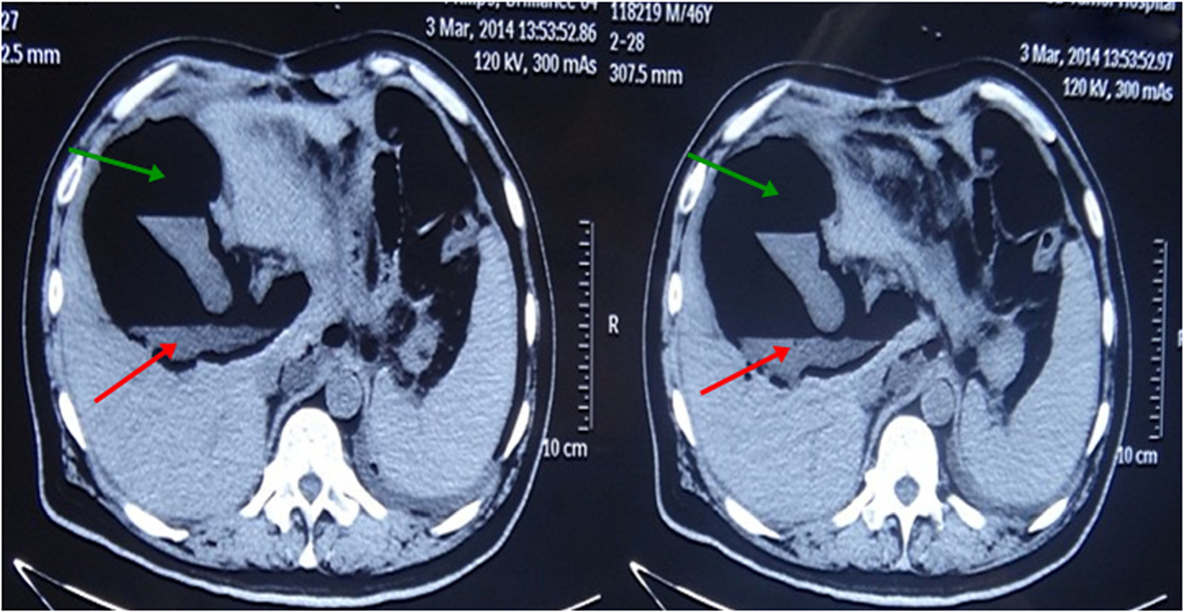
CT scans investigation. CT abdomen/pelvis showing fluid (red arrow) and gas (green arrow) in the liver, gallbladder cannot be detected
